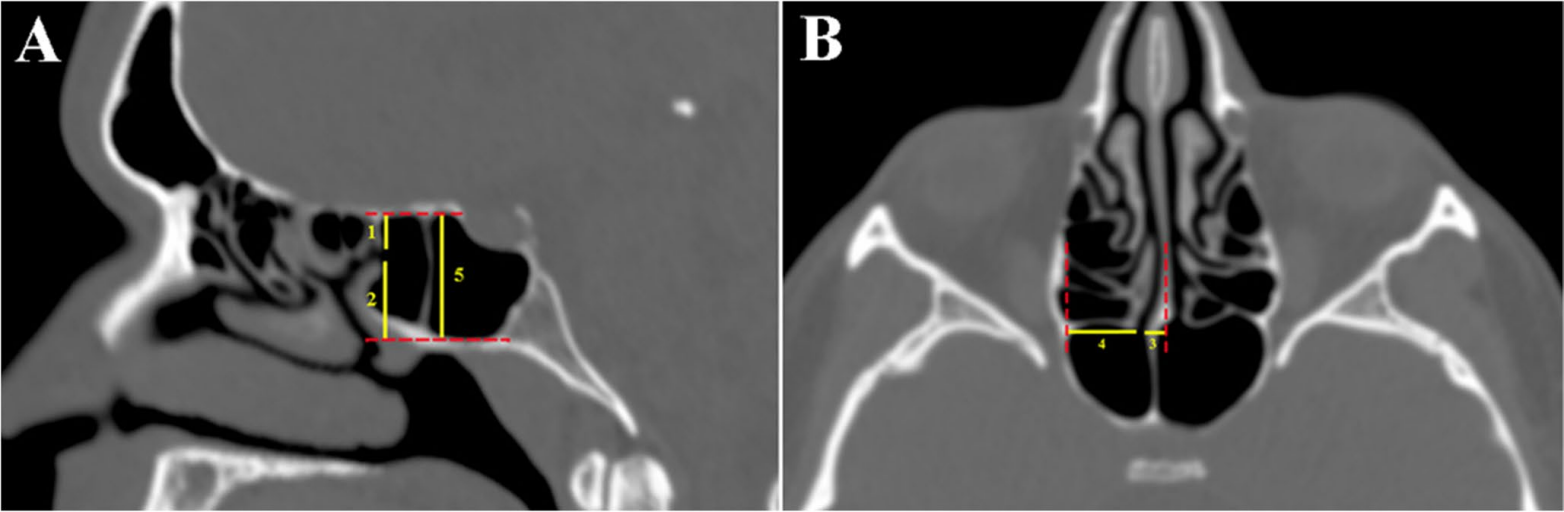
(A) Representative sagittal CT image showing three vertical measurements of the sphenoid sinus ostium (SSO): *Line 1*: vertical distance from the superior margin of the SSO to the roof of the sphenoid sinus. *Line 2*: vertical distance from the inferior margin of the SO to the floor of the sphenoid sinus. *Line 5*: total height of the sphenoid sinus (roof to floor). Red dotted lines indicate the superior and inferior boundaries of the sphenoid sinus, aligned with the corpus sphenoidale. (B) Representative axial CT image showing two horizontal measurements of the SSO: *Line 3*: distance from the medial margin of the SSO to the midline of the nasal septum (rostrum width). *Line 4*: distance from the lateral margin of the SSO to the lateral border of the anterior wall of the sphenoid sinus. Red dotted lines indicate the midline of the nasal septum and the lateral boundary of the sphenoid sinus
